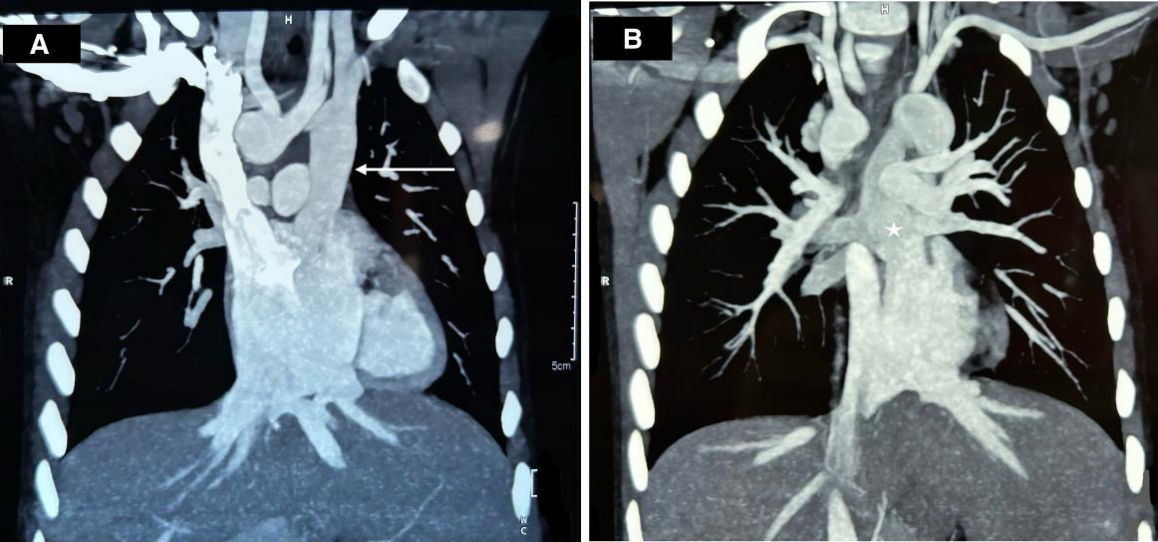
Cardiac CT of the patient showing (*A*) LSVC draining into the left side of the common atrium (arrow) and (*B*) pulmonary veins draining into the LSVC (star).
